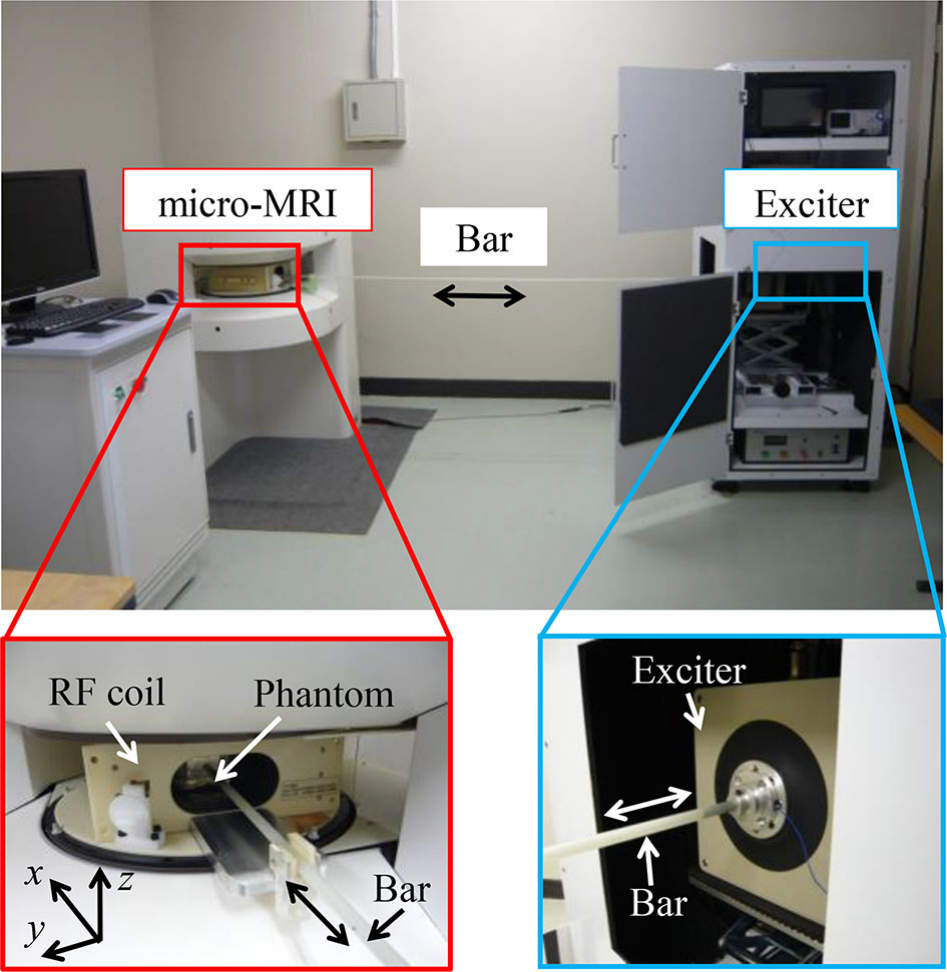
Experimental arrangement for MRE with micro MRI
